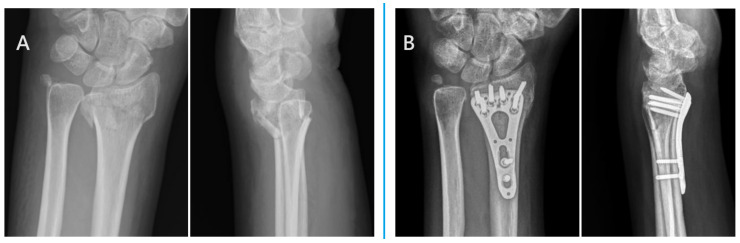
Type C1 fracture. (A) Pre-operative X-ray and (B) post-operative X-ray.
